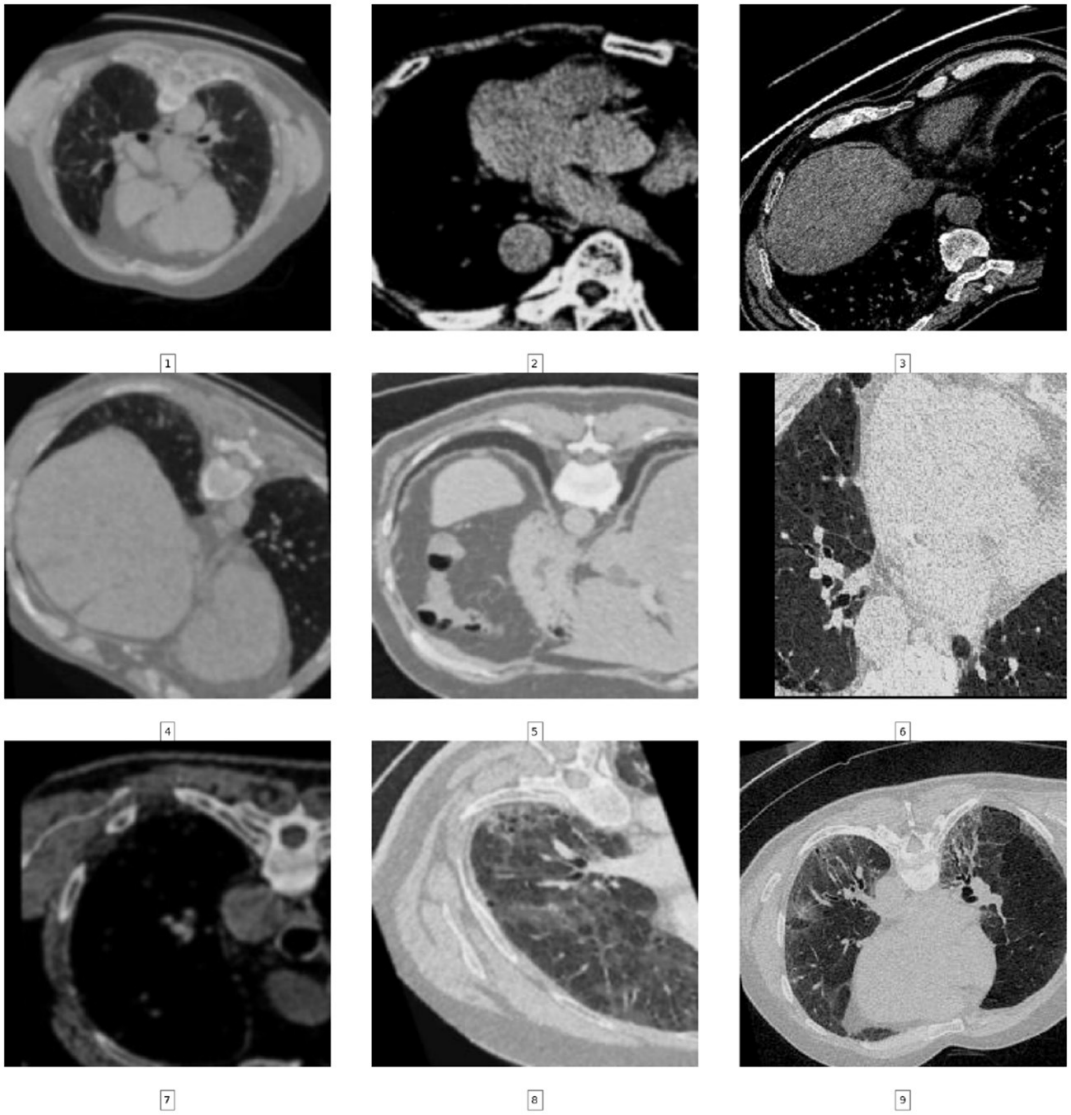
Examples of the CT images with applied augmentation techniques.
